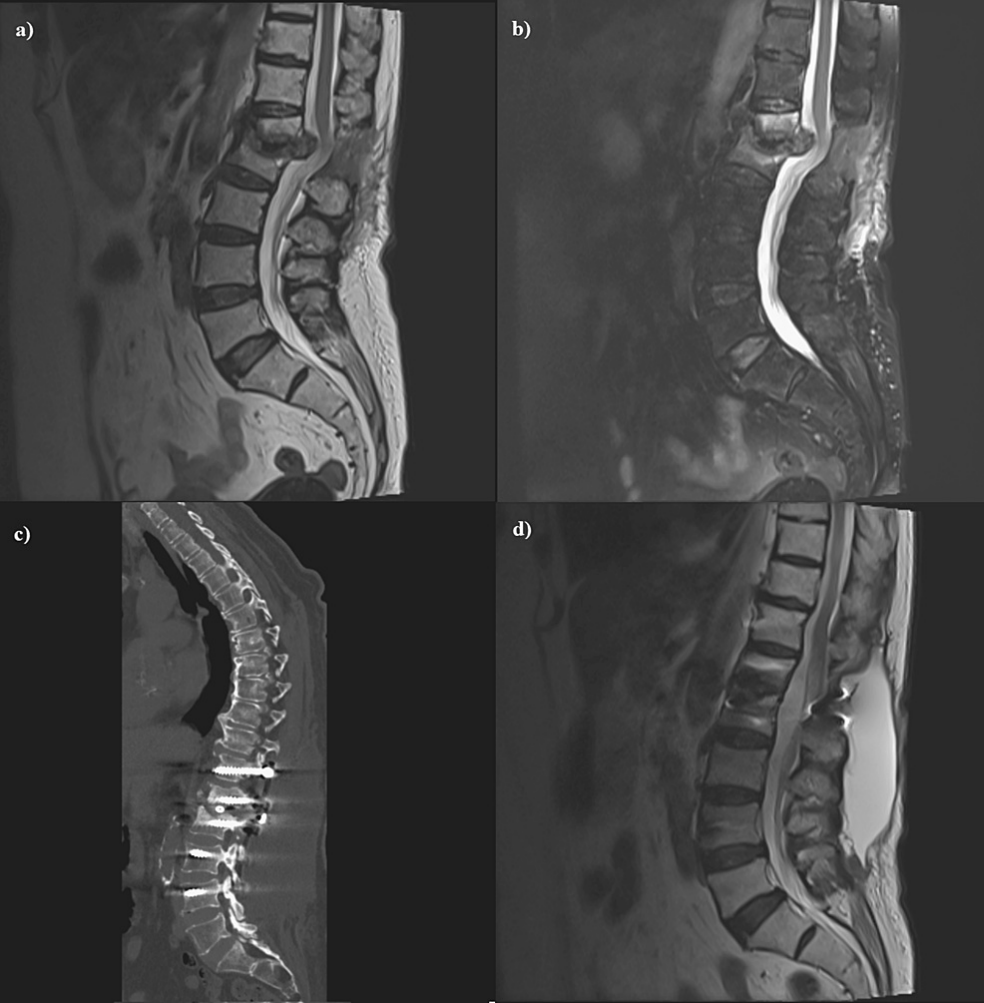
A 66-year-old female presenting with L1-L2 spinal brucellosis. (a and b) Sagittal T1 and T2-weighted MRI images showing lesions involving L1-L2 vertebral bodies and intervertebral discs. Epidural abscess and inflammatory granuloma formation. (c) Postoperative CT shows posterior interbody fusion and bilateral transpedicular titanium screws. (d) Six-month postoperative T2-weighted MRI images.
